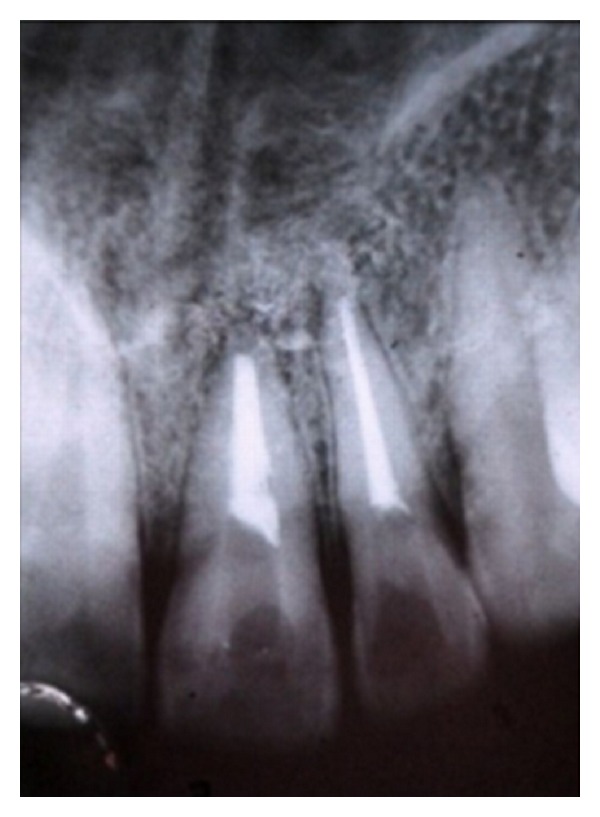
Postoperative radiograph after 3 months.
